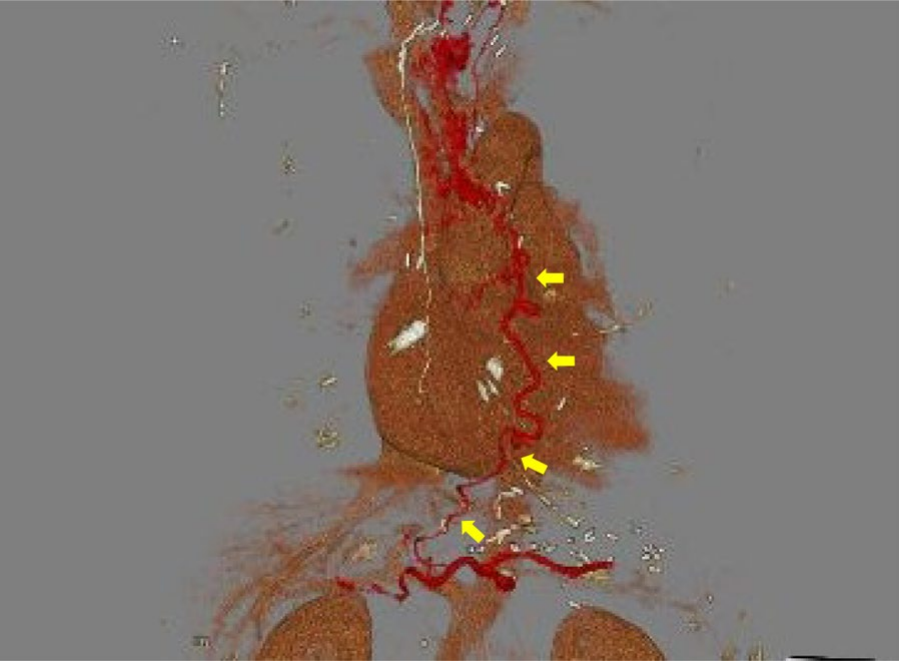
3D-CT angiography findings. The dominant region of the RGEA was distributed to the upper part of the gastric tube
